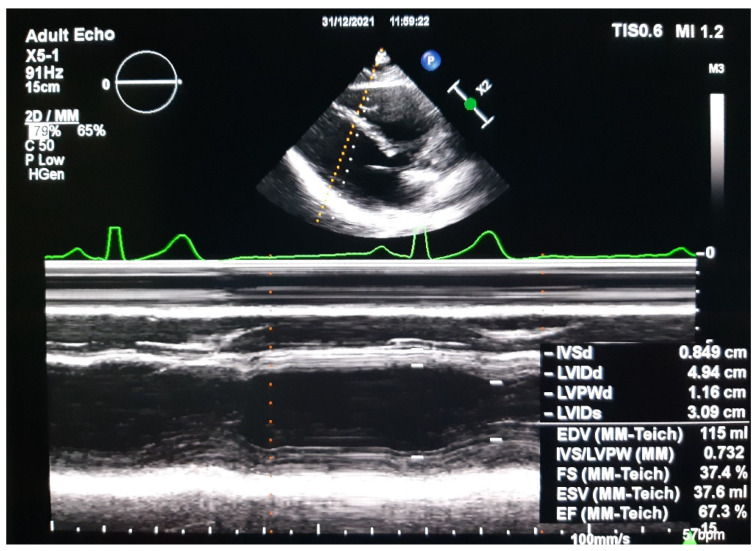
M-Mode echocardiography of a healthy pregnant patient, in parasternal long-axis view showing left ventricular fractional shortening and ejection fraction–IVSd (interventricular septum thickness at diastole, LVIDd (left ventricle internal diameter during diastole), LVIDs (left ventricular internal diameter during systole, LVPWd (left ventricular posterior wall thickness during diastole, EDV (end diastole volume), ESV (end systole volume), EF (ejection fraction), FS (fractional shortening). Courtesy of Elias Cardiology Department.
